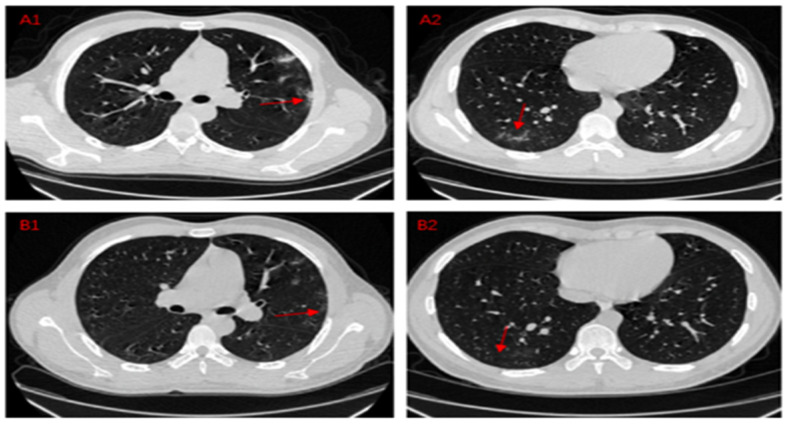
CT scan image of a COVID-19 pneumonia patient [36].
